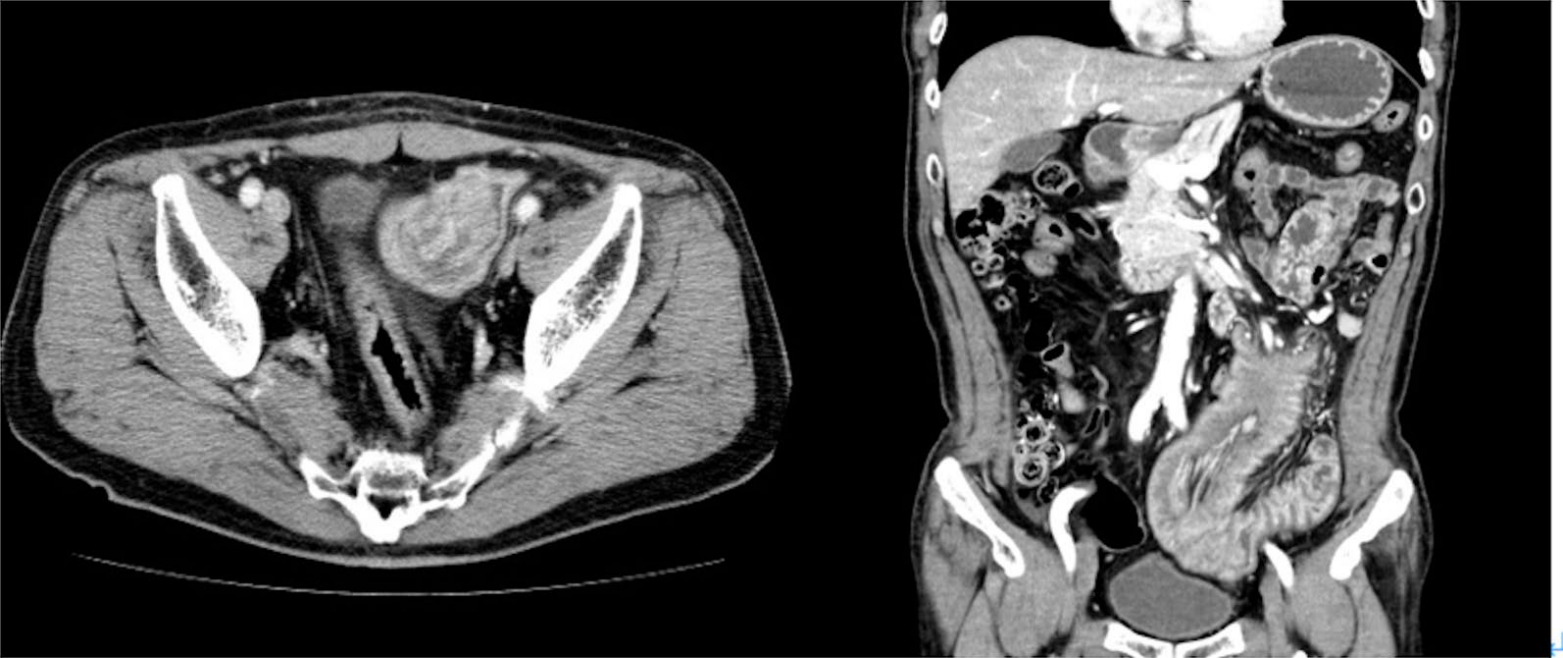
Abdominal contrast-enhanced computed tomography. The image shows a 20-cm-long small bowel–small bowel type superimposed findings with a preceding mass with sparse contrast in the jejunum. No apparent pancreatic abnormalities were observed
